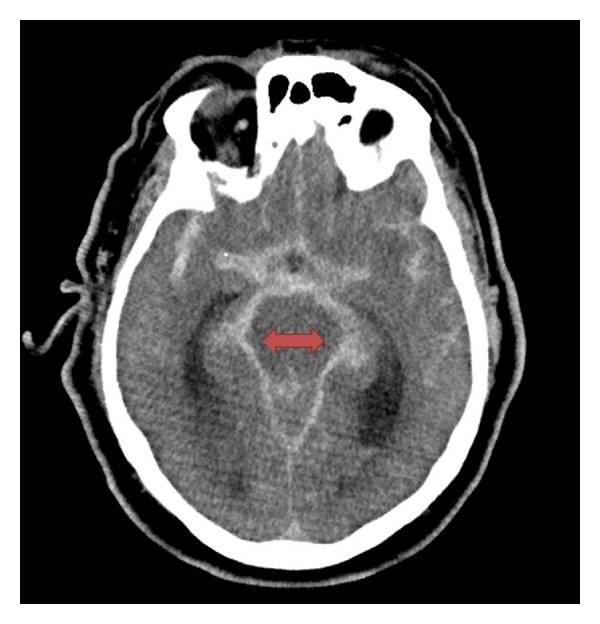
Hospital day 3 CT head without contrast: Diffuse subarachnoid blood products. There are also blood products layering within the occipital horns of the bilateral lateral ventricles. There are also subarachnoid blood products extending inferiorly surrounding the cervical cord. This is consistent with rupture of the patient's known basilar artery aneurysm. The ventricles are further enlarged with findings of transependymal CSF migration. There is no herniation.
